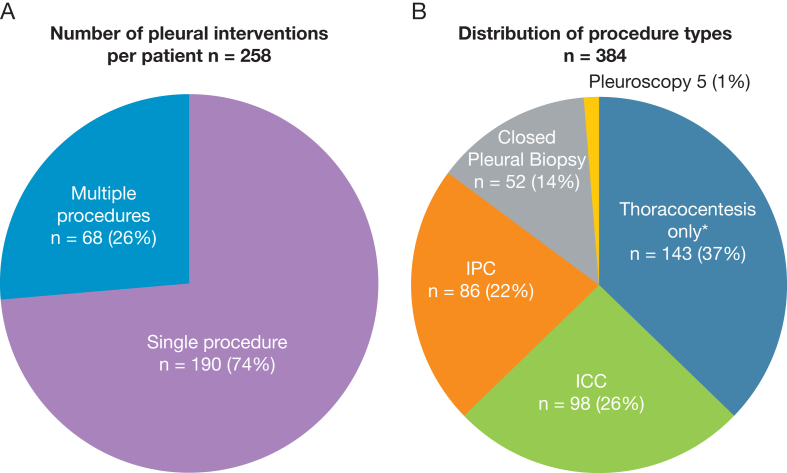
A, Number of pleural interventions per patient. B, Type of pleural interventions. Of the 258 patients, 16 patients had combined IPC and closed, percutaneous, ultrasound-guided closed pleural biopsy. ∗Thoracocentesis only: no other pleural intervention (eg, ICC or IPC insertion) was performed concurrently. ICC = intercostal catheter; IPC = indwelling pleural catheter).
